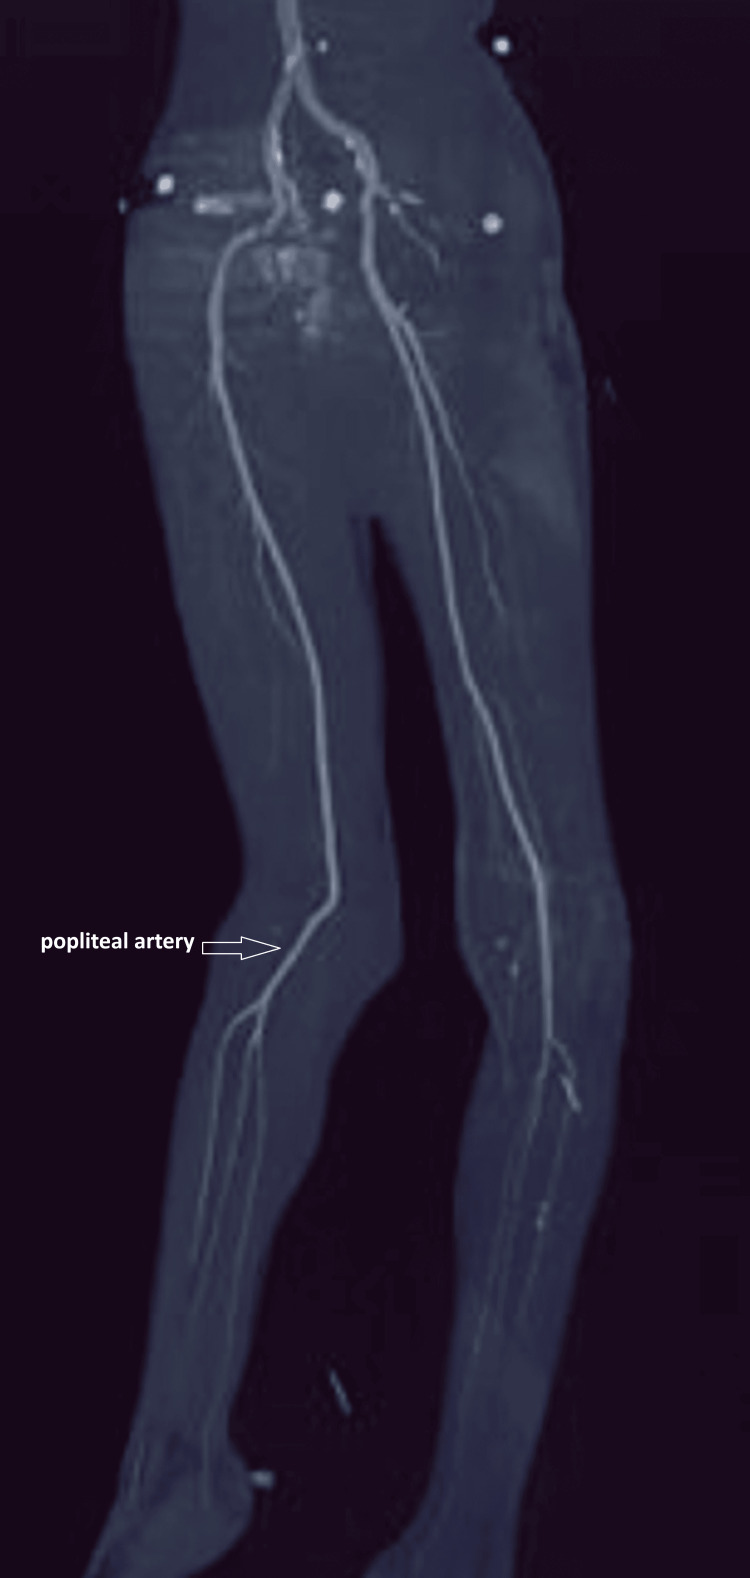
CT angiography of the lower limbs demonstrating preserved arterial patency despite traumatic knee dislocation The popliteal arteries are clearly visualized along their course, with no evidence of intimal injury, thrombosis, dissection, or contrast extravasation, highlighting that normal distal pulses do not exclude the need for vascular imaging in high-energy knee dislocations
